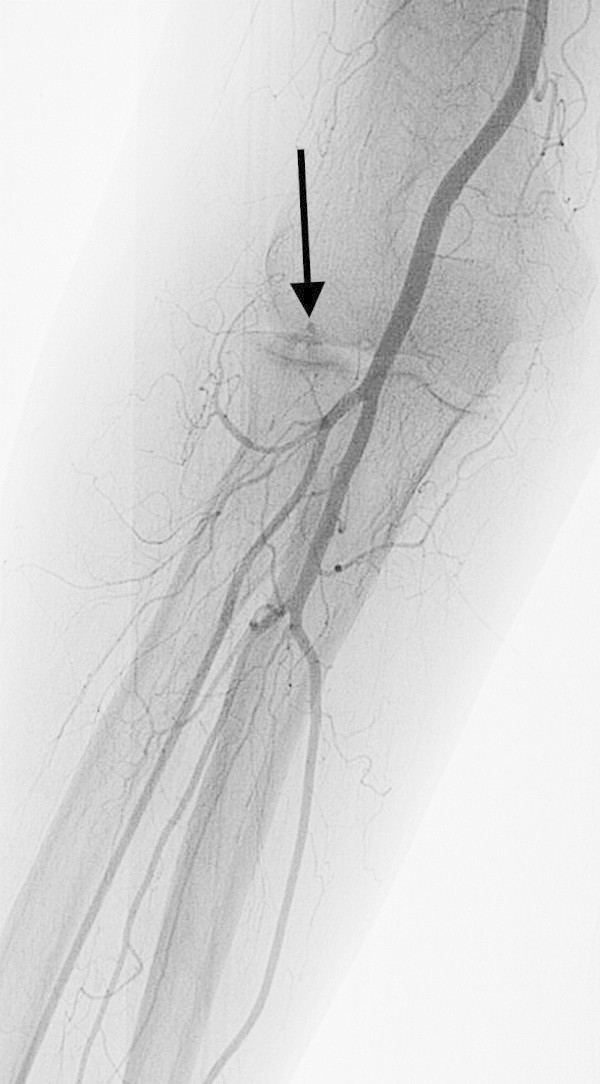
Damage to the recurrent artery caused by the guide wire in a 72-year-old woman with a left vestibular Schwannoma. Resistance was felt to wire operation for sheath insertion, and fluoroscopy showed that it was caught in the recurrent artery in the elbow region. After repeated reinsertions, the wire was successfully inserted into the brachial artery, and the sheath could be inserted. Subsequent contrast showed extravasation (arrow) and a subcutaneous hematoma, but manual compression prevented the hematoma from expanding. Ultrasound scanning at a later date confirmed that there was no formation of pseudoaneurysm or arteriovenous fistula. The damage probably occurred when resistance was felt during insertion of the wire. This type of situation can be expected if the wire is forcibly inserted into the recurrent artery, and treatment must be applied to ensure that the hematoma does not become too large.
